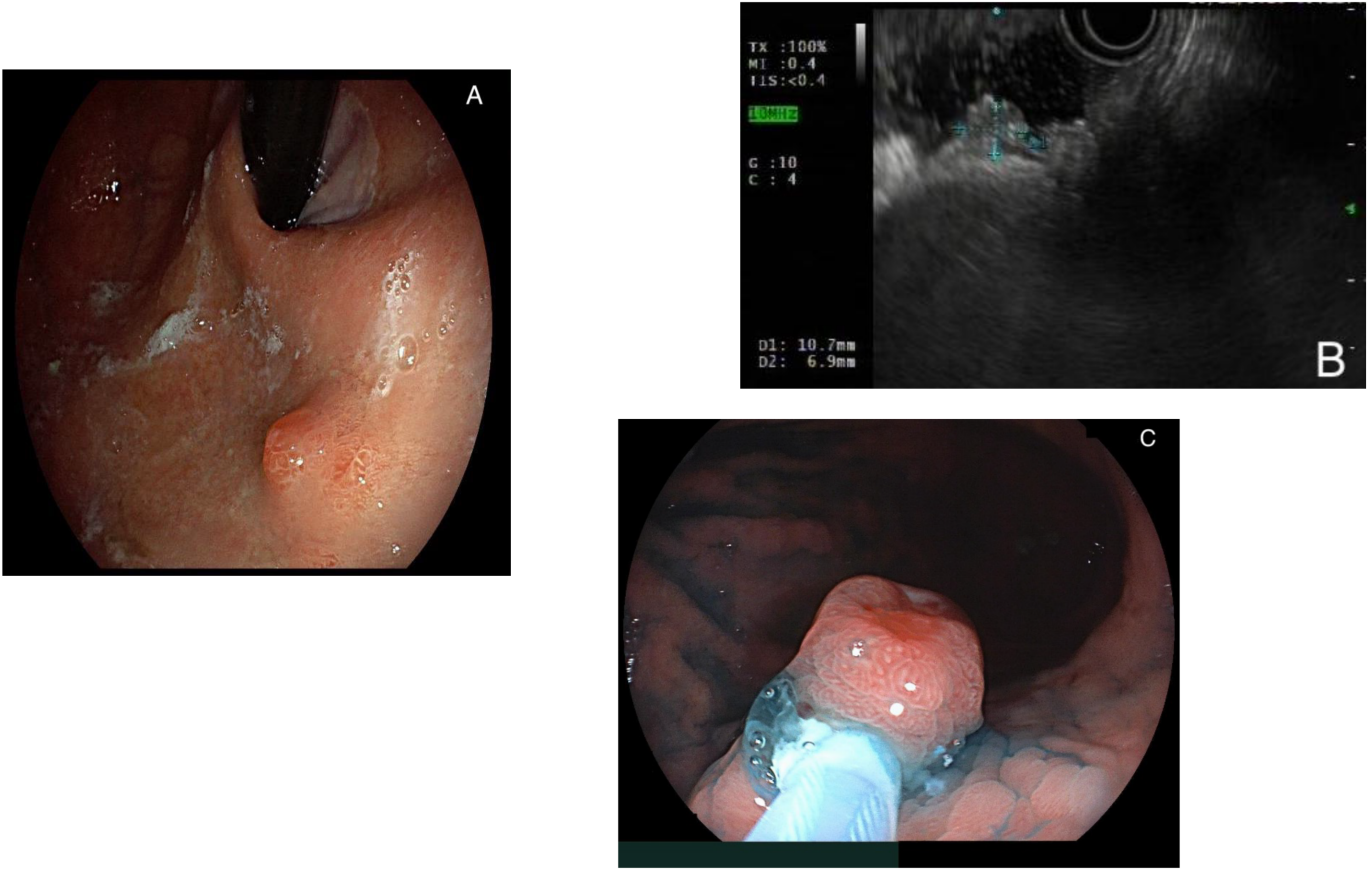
(A) Endoscopic appearance of a gastric neuroendocrine tumor (gNET), visualized in retroversion; (B) endoscopic ultrasound aspect of a gNET; (C) endoscopic resection via hot- endoscopic mucosal resection (EMR) of a gNET.
